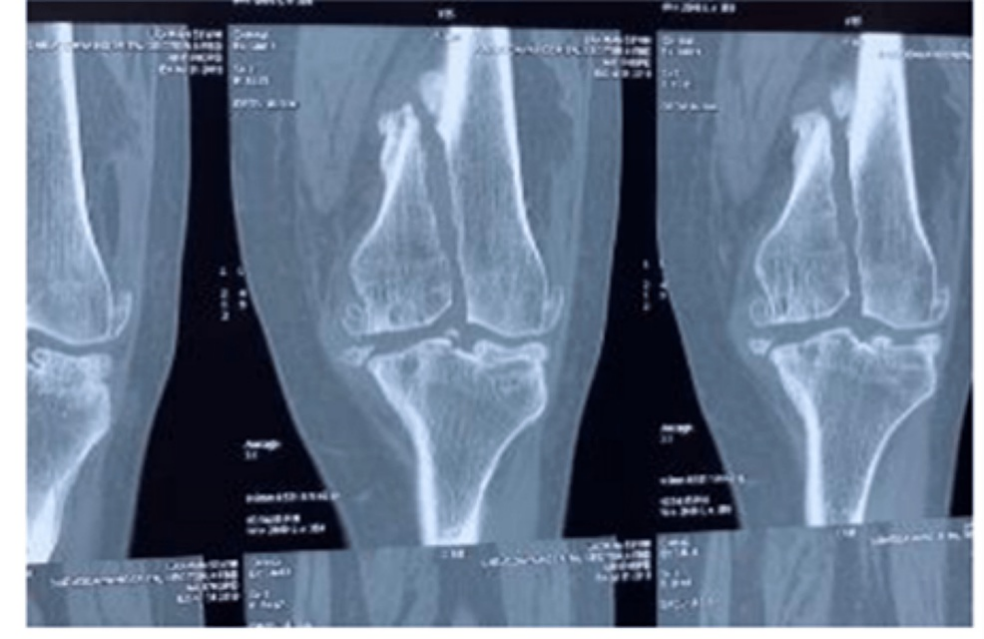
CT Scan (coronal cuts) images of the left knee showing distal femur stress fracture with intra-articular extension.
